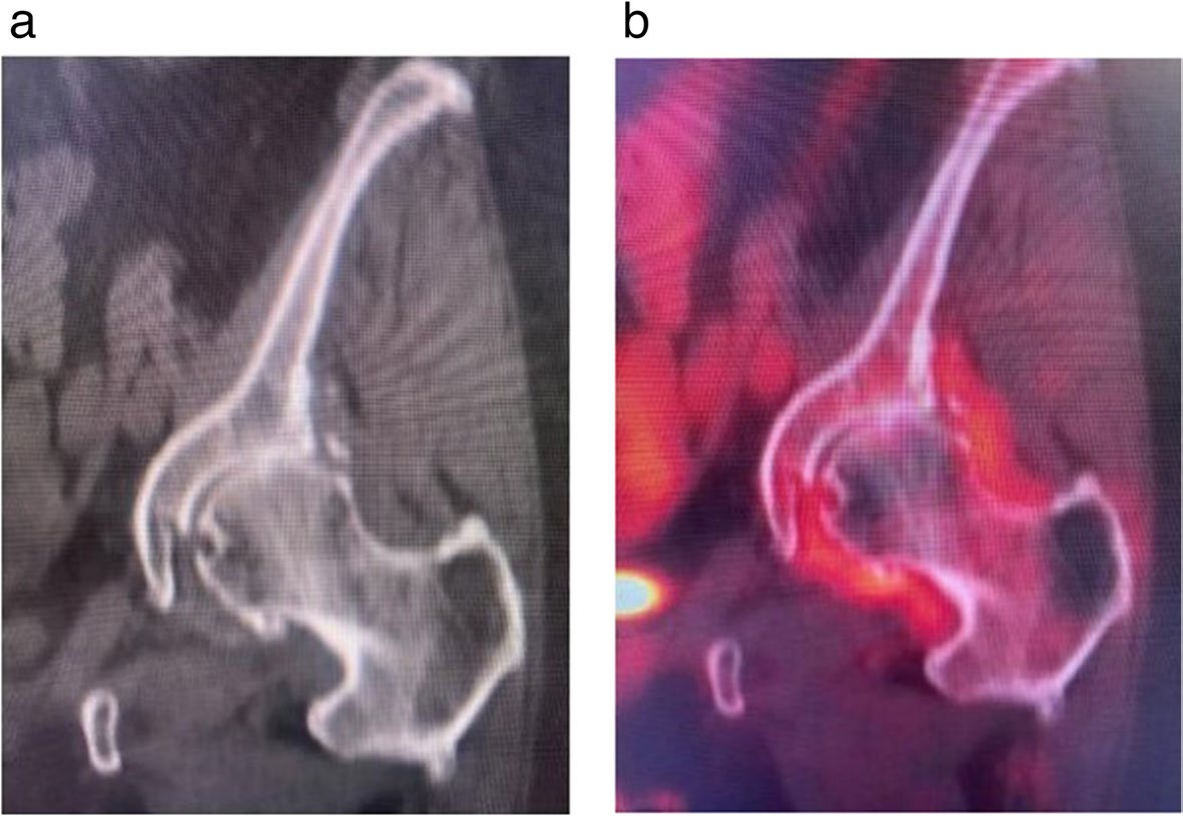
^18^F-FDG uptakes around left hip with osteoarthritis. a Coronal CT image of the left hip demonstrates joint space narrowing along the superior aspect of the left hip joint, compatible with mild-to-moderate OA. b Fused coronal ^18^F-FDG PET-CT image of the left hip shows hypermetabolic activity along the periphery of the left hip joint in the expected location of the synovium and joint capsule, compatible with synovitis associated with OA
